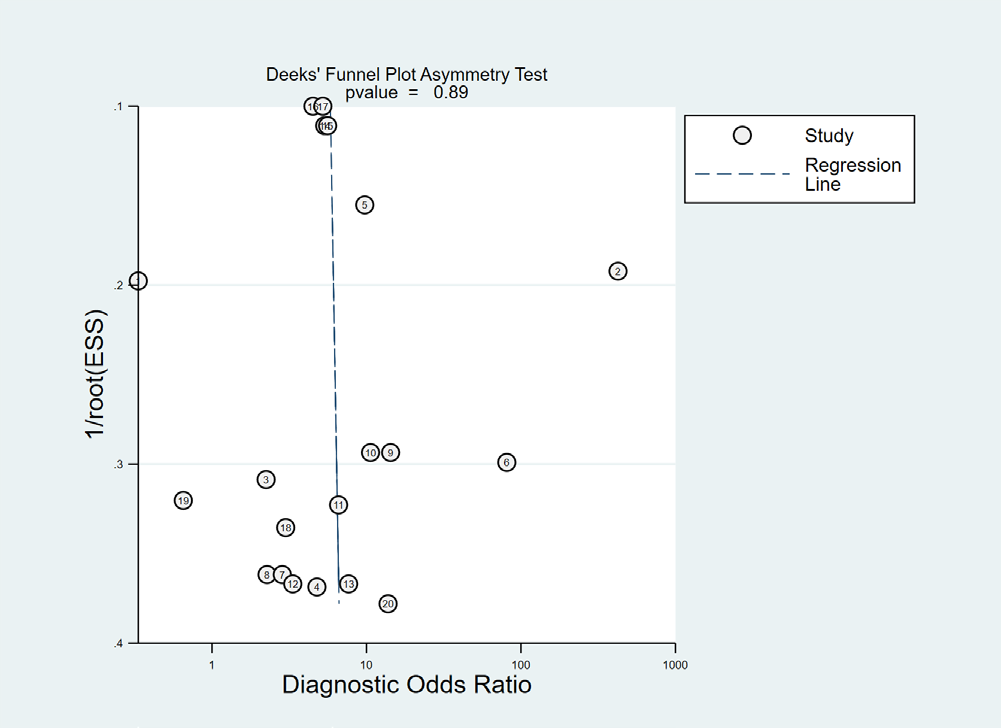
Funnel plot of MRI for the diagnosis of ALT. ALT = acetabular labral tears, MRI = magnetic resonance imaging.
